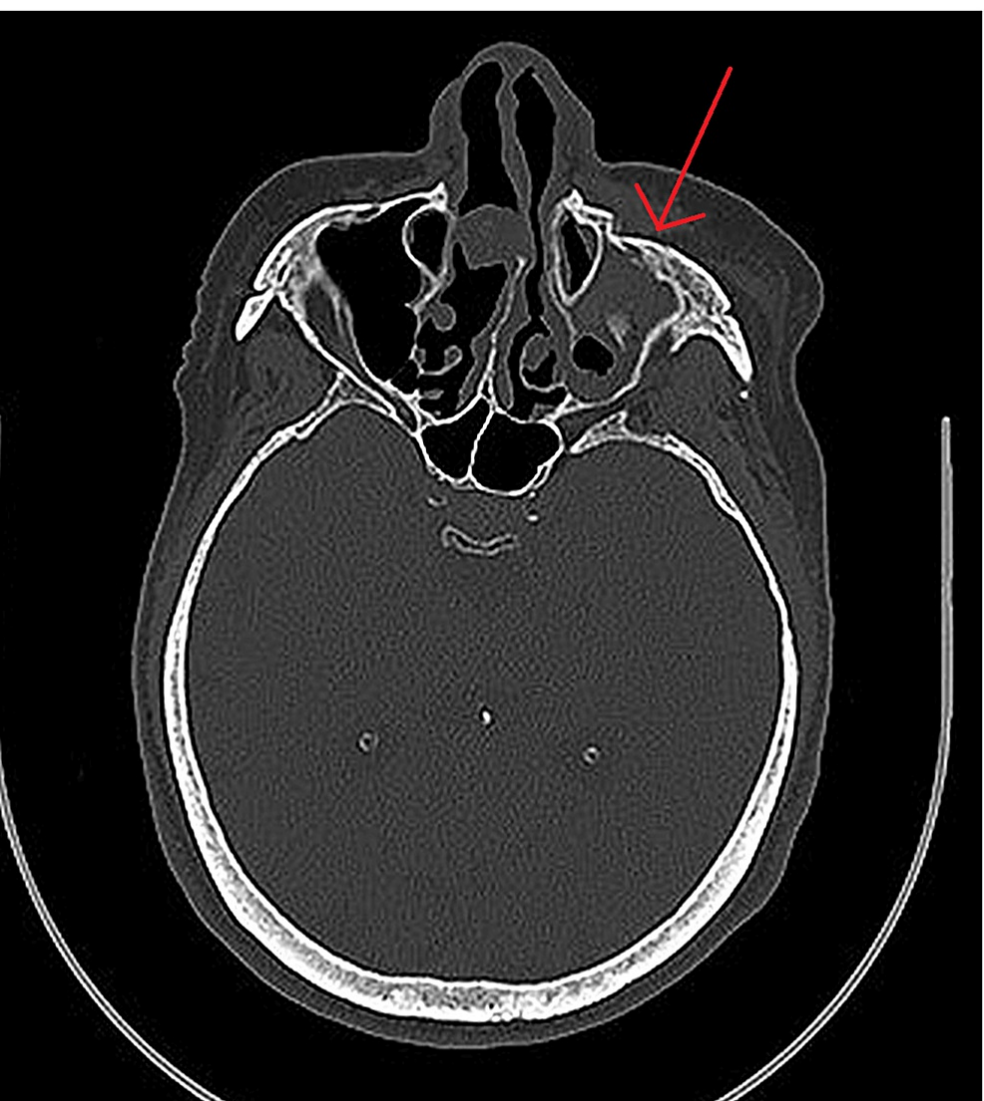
Preoperative CT scan Red arrow: left zygomatic-maxillary complex fracture
